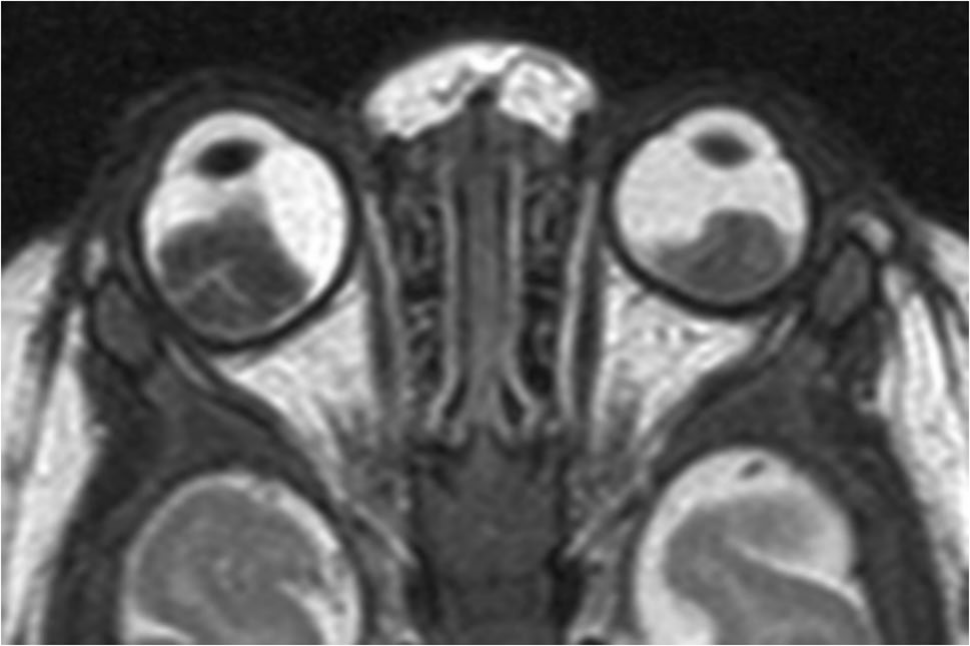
Bilateral retinoblastoma. Axial T2-weighted image of the orbits demonstrates intraocular hypointense masses arising from the retina of both eyes
